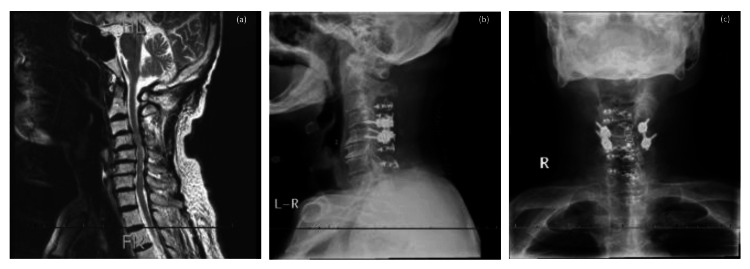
Findings on imaging before and after surgery. (a) Preoperative sagittal magnetic resonance image obtained 37 days before surgery showing cervical kyphosis with spinal canal stenosis, which was particularly evident at the C4/5 level. The patient subsequently underwent laminoplasty at C3-C7, posterior spinal fusion at C4/5, and foraminotomy at C5. (b) Sagittal and (c) frontal radiographs obtained on postoperative day 14 confirm screw insertion into the C4/5 pedicles and rod fixation.
